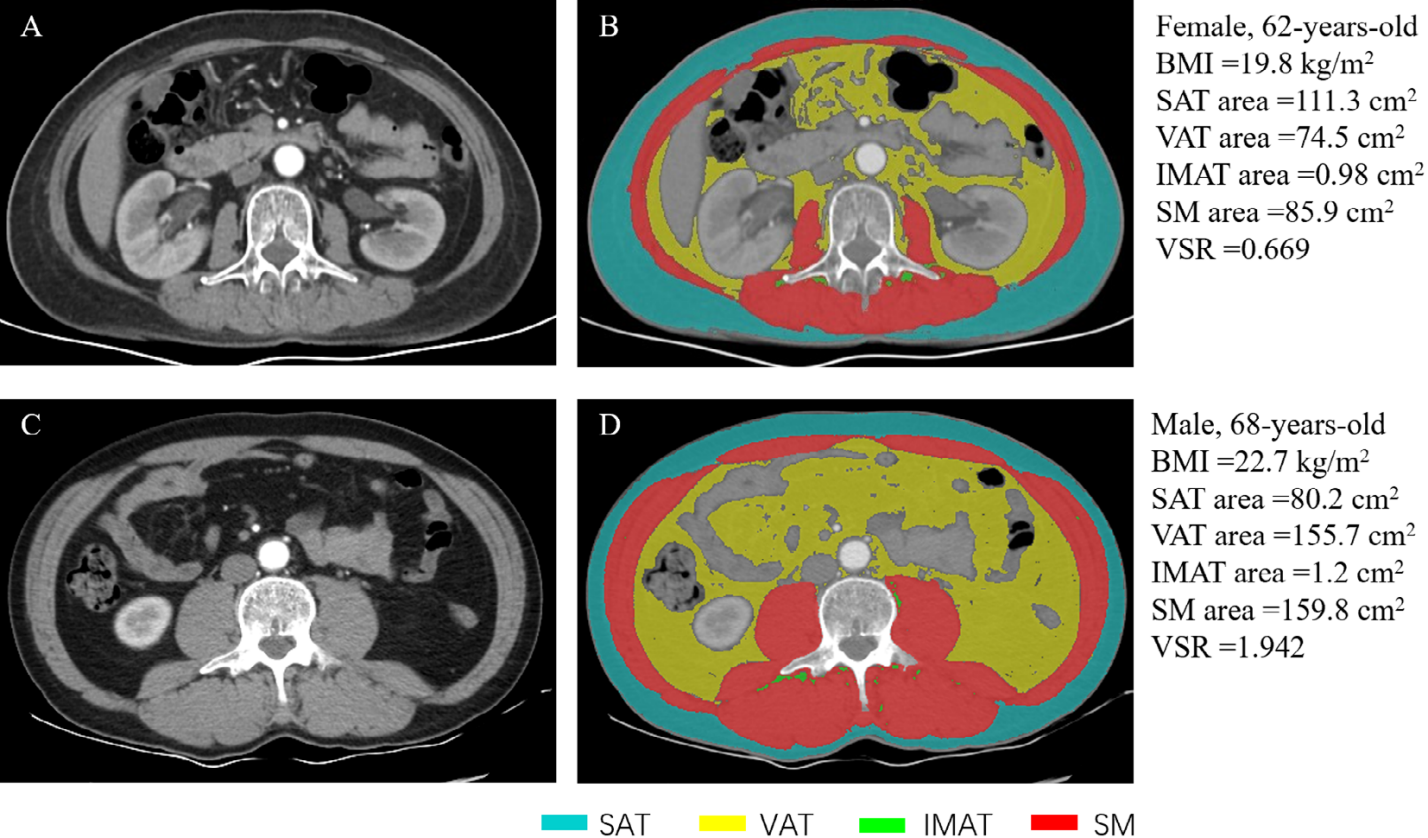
Cross‐sectional CT images at the third lumbar vertebra level used to measure body composition variables. BMI, body mass index; IMAT, intramuscular adipose tissue; SAT, subcutaneous adipose tissue; SM, skeletal muscle; VAT, visceral adipose tissue. (A, B) Female, 62 years old; (C, D) male, 68 years old.
